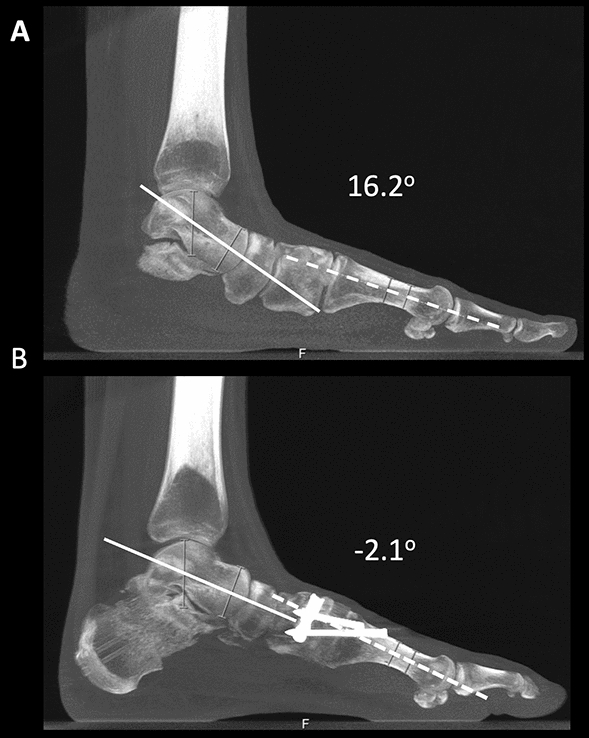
Example of preoperative (A) and postoperative (B) measurements of weight-bearing CT sagittal plane talus-first metatarsal angle (TFMA) in a progressive collapsing foot deformity patient is demonstrated. The preoperative TFMA was 16.2° of collapse of the medial longitudinal arch (plantar vertex). It was corrected postoperatively to 2.1° of dorsal apex (negative value), with a total correction of 18.3°
